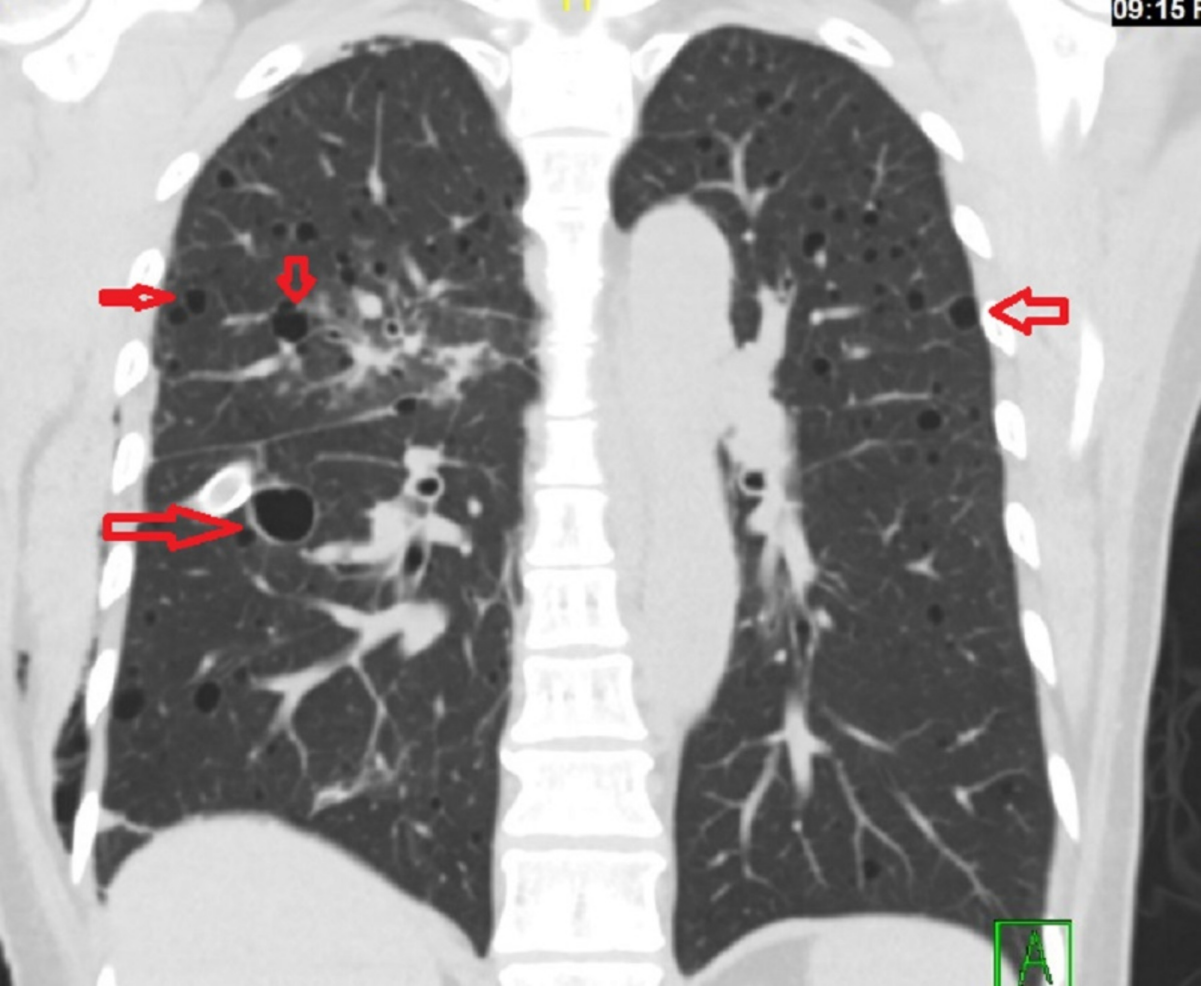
Coronal section: Chest computed tomography scan showing multiple cysts
